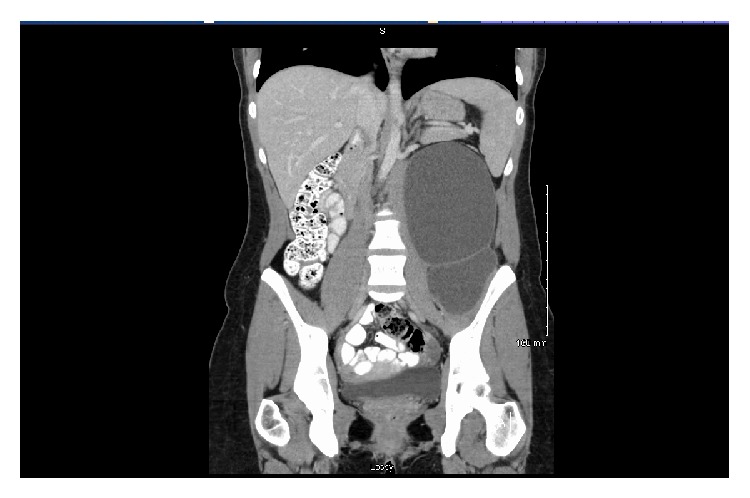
Computed tomographic scan showing a large retroperitoneal mass with cystic and solid components.
